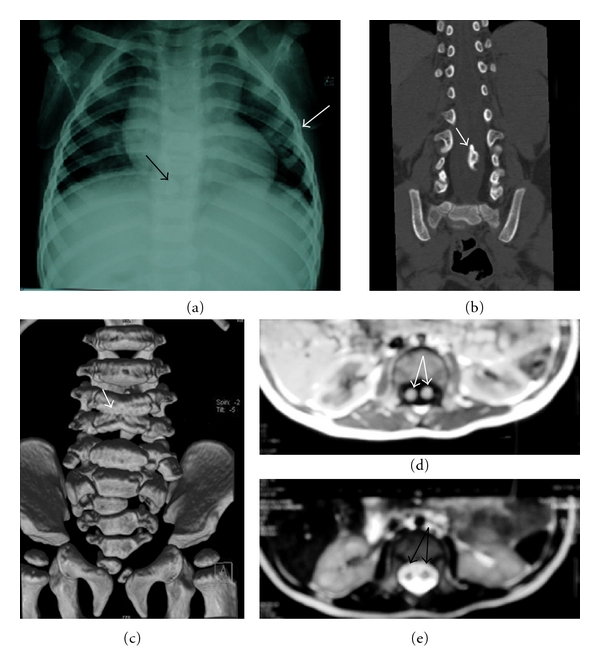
Plain radiographs revealed cleft vertebrae of the ninth thoracic vertebrae, and bifid rib of the fourth rib (Figure 2(a)). Computed tomography (CT) scans showed split cord malformations, block vertebrae of the third and fourth vertebrae, and hemivertebra of the second sacral vertebrae (Figures 2(b) and 2(c)). Magnetic resonance imaging (MRI) showed split cord malformations (Figure 2(d) and 2(e)).
